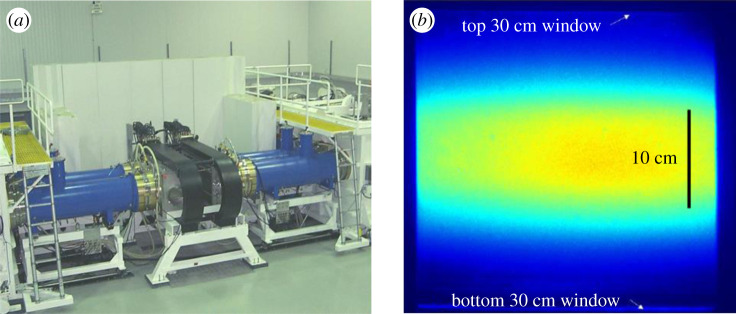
(*a*) Photo of the Electra 30-cm aperture electron beam-pumped amplifier before the x-ray shielding was installed. Two coaxial transmission lines using a water dielectric on each side of the laser cell provide a 500 kV pulse to the cathodes. (*b*) The vertical dimension of Electra's cathode was reduced from 30 cm to 10 cm to provide higher e-beam pump intensity for ArF operation. The photo shows a time resolved image of the emitted light produced by the reduced size electron beams interacting with the laser gas looking along the laser axis. The gate is 10 ns and is taken at the time of peak current. The vertical black line is shown for scale. (Online version in colour.)
